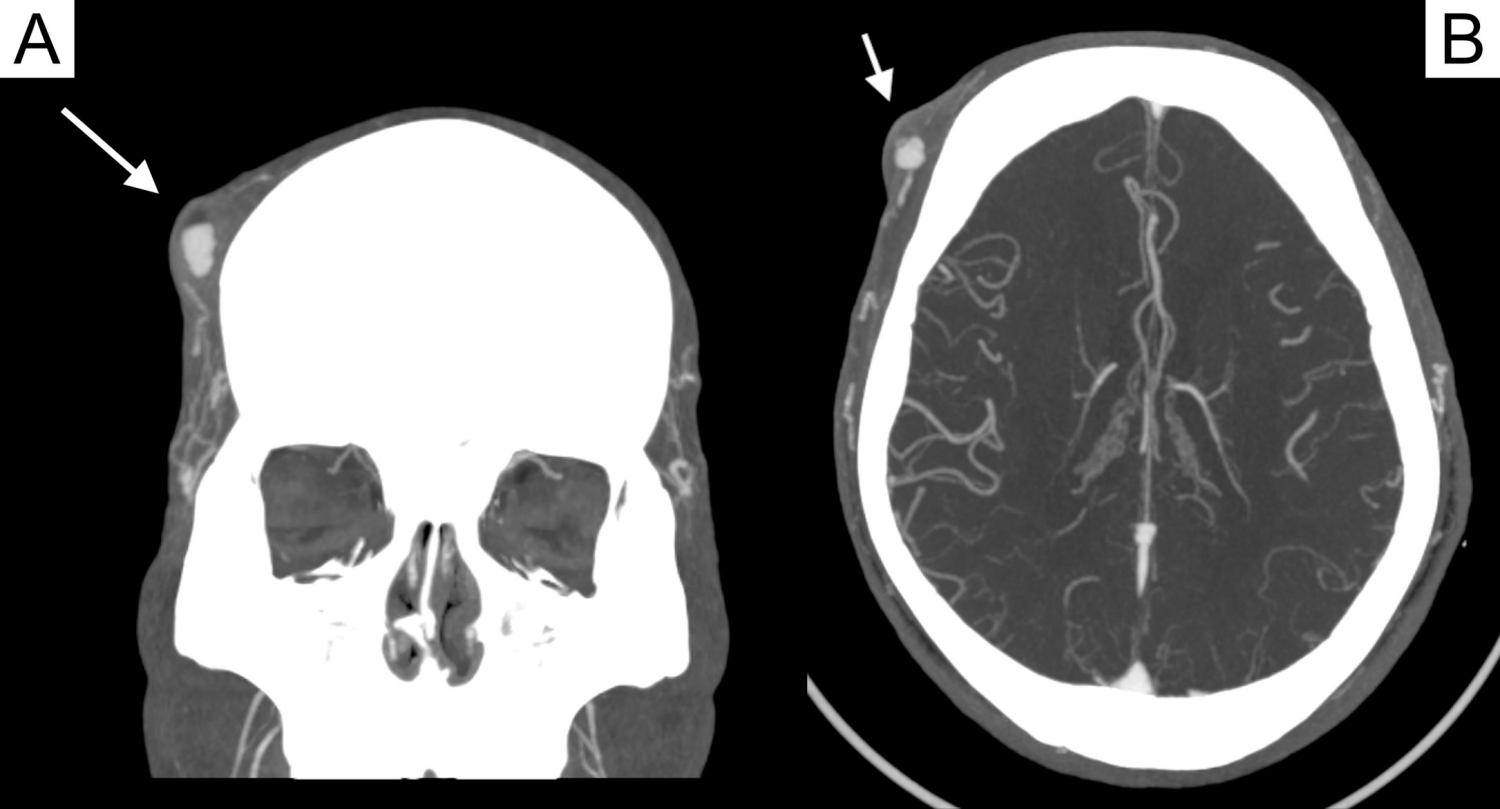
Coronal (A) and Axial (B) Head CT-Angiogram with 7mm round pool of contrast (white arrows) supplied by an artery with a questionable draining vein, consistent with an AVM or less likely pseudoaneurysm.
